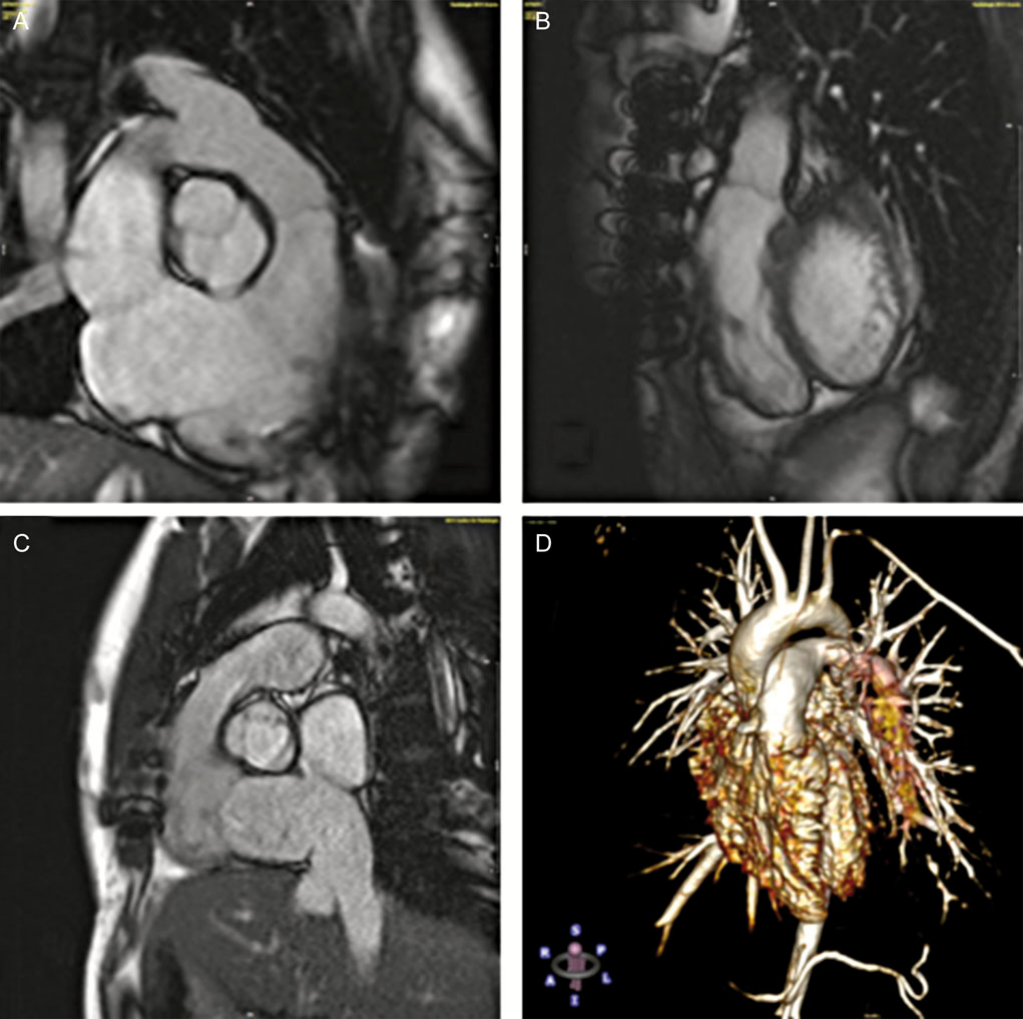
Cardiac magnetic resonance imaging examples of DPH. (A) Coronary three-chamber view at diastole 72 months after DPH implantation in a 20-year old patient; (B) sagittal view of the patient (A) at diastole; (C) sagittal view of DPH at systole 78 months after implantation in a 24-year old patient; (D) contrast-enhanced angiography 116 months after DPH implantation in a 10-year old patient.
